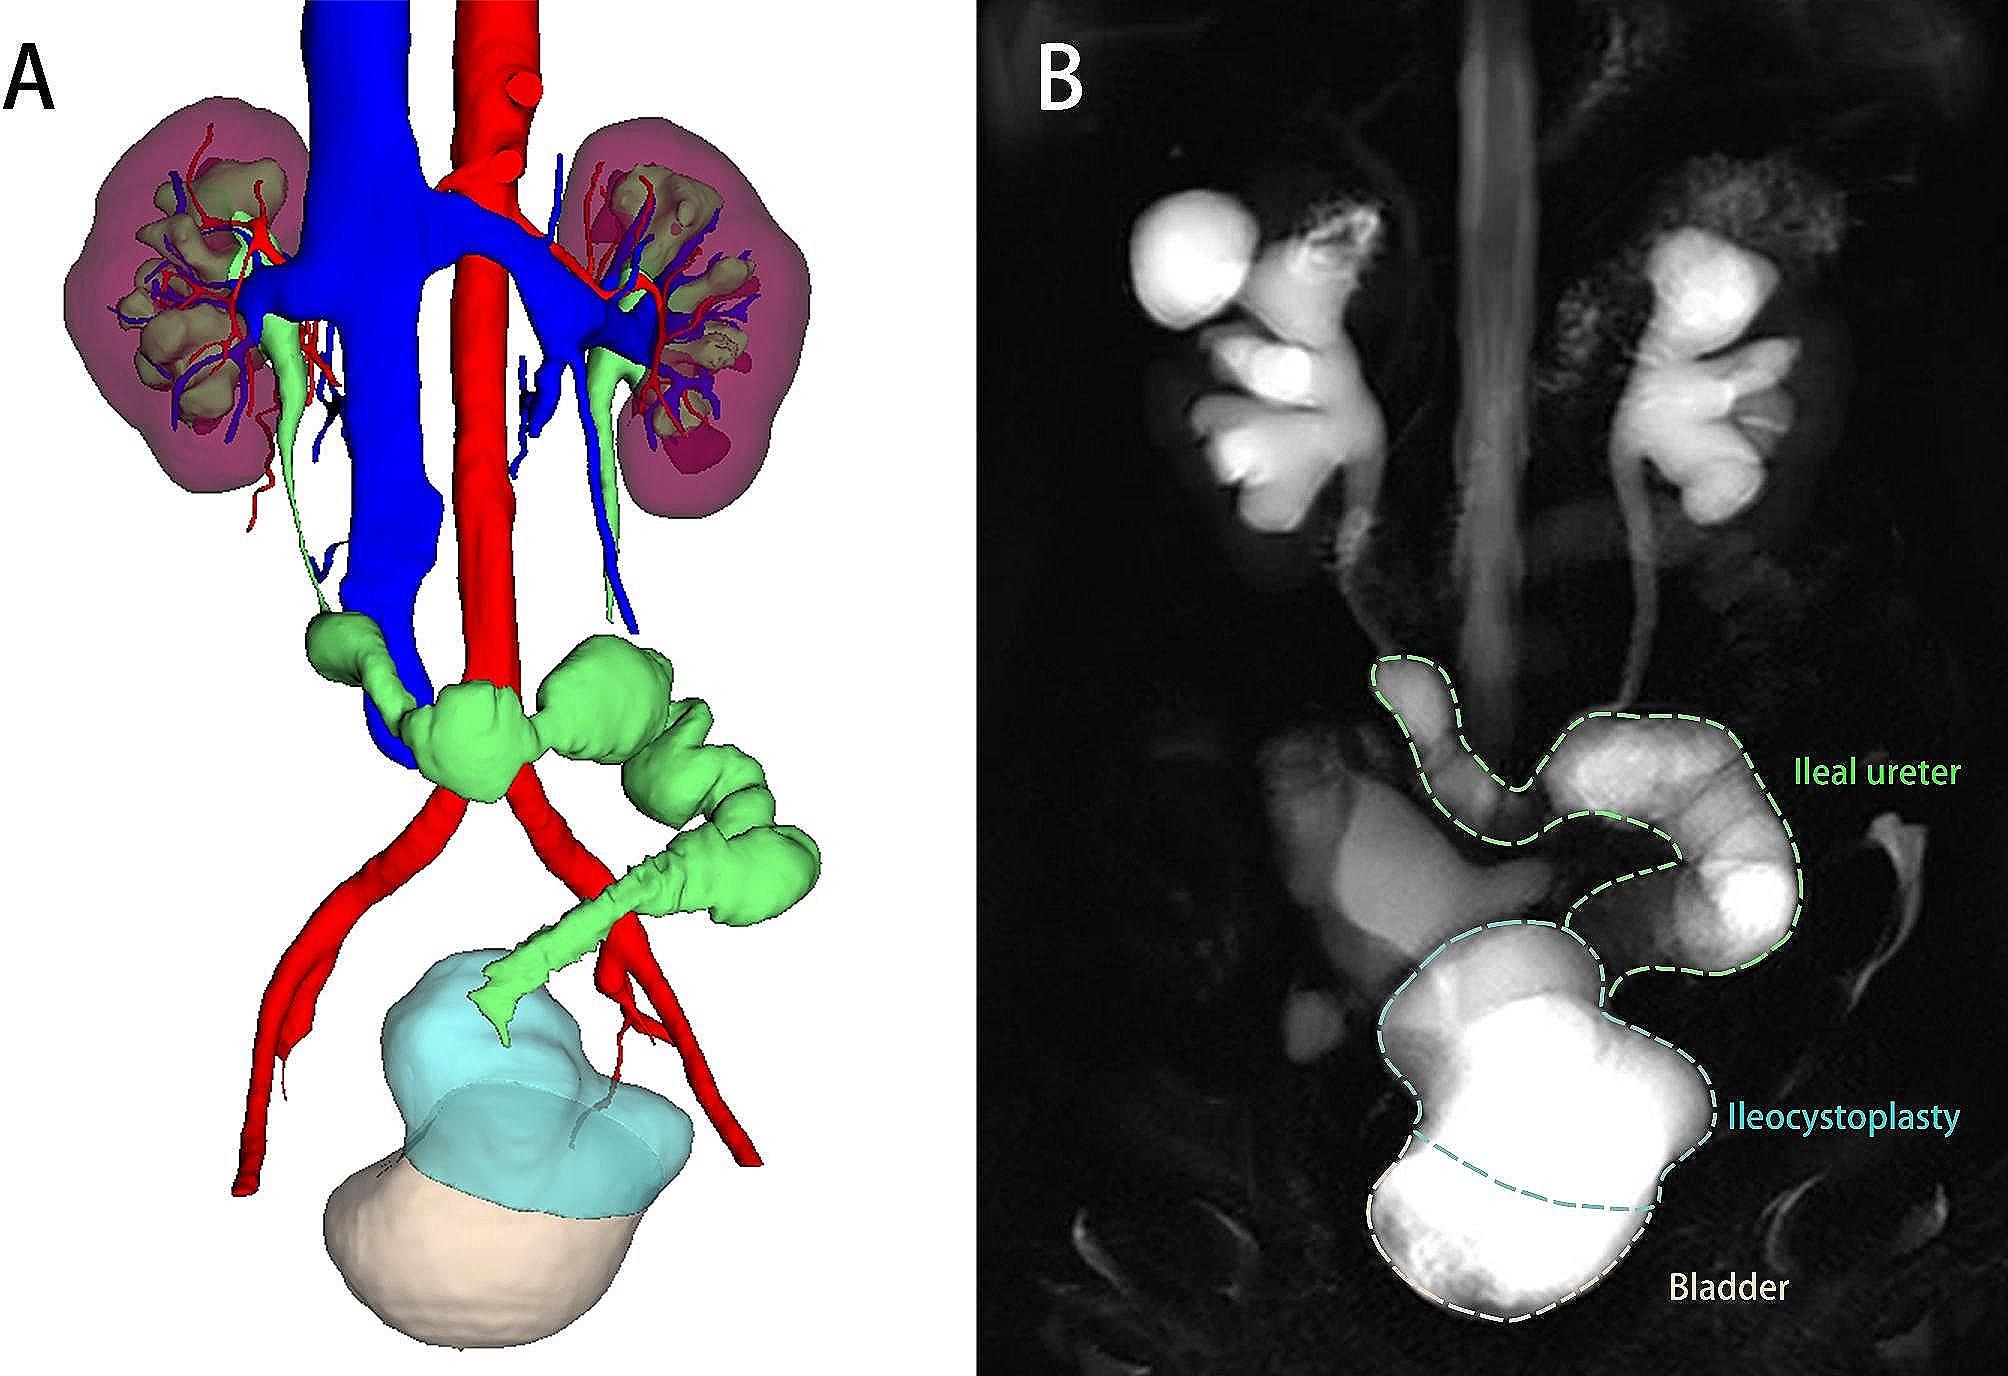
Postoperative radiographic examination during follow-up. (A) Postoperative three-dimensional CTU (B) Functional cine MRU
